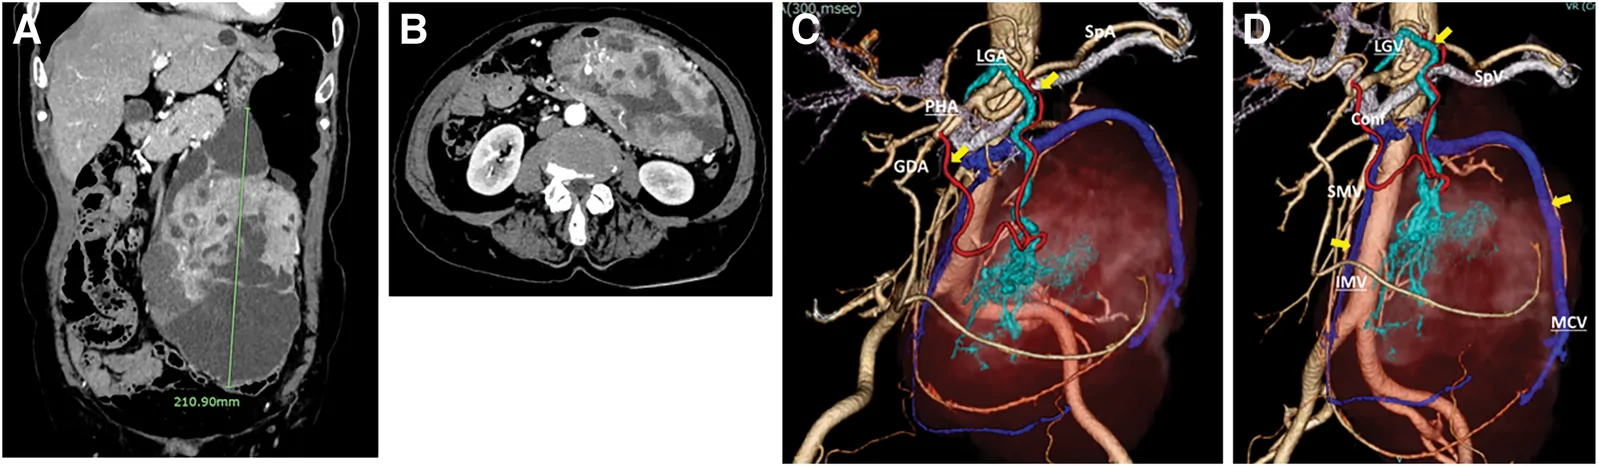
Contrast-enhanced CT findings of the tumor. (A) Contrast-enhanced CT shows a large intra-abdominal mass measuring 21 cm in diameter. (B) A small aneurysmal structure is identified within the tumor. (C) Arterial-phase image demonstrating tumor supply from the left gastric and proper hepatic arteries, indicated by yellow arrows. (D) Venous-phase image showing tumor drainage into the left gastric, middle colic, and inferior mesenteric veins, indicated by yellow arrows. Conf, confluence of the superior mesenteric vein and splenic vein; GDA, gastroduodenal artery; IMV, inferior mesenteric vein; LGA, left gastric artery; LGV, left gastric vein; MCV, middle colic vein; PHA, proper hepatic artery; SMV, superior mesenteric vein; SpA, splenic artery; SpV, splenic vein
